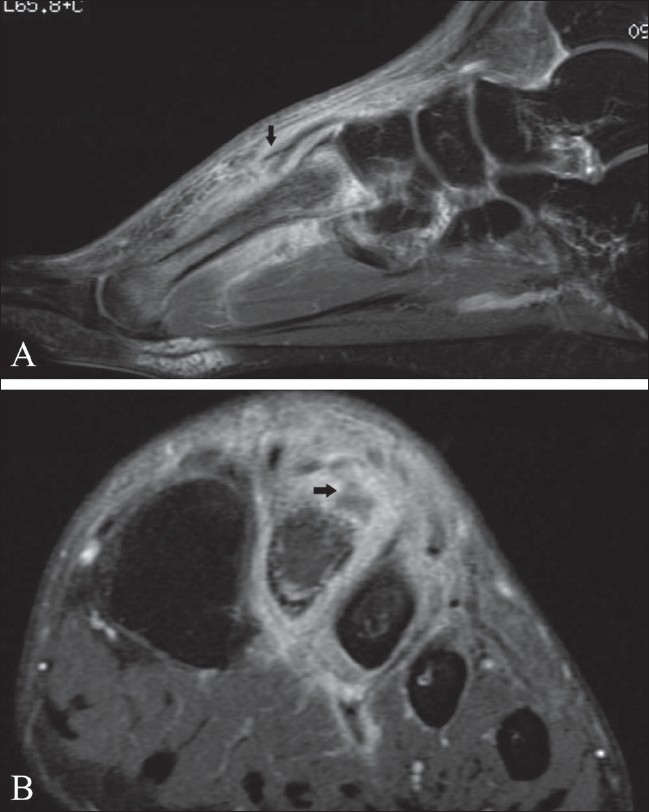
Tuberculous osteomyelitis of the second metatarsal bone of the left foot. Sagittal, fat-suppressed, T2W MRI (A) shows bone marrow edema, adjacent tenosynovitis (arrow) of the extensor tendon and increased signal within the adjacent soft tissues on the plantar and dorsal aspects of the foot. Coronal, contrast-enhanced, fat-suppressed, T1W MRI (B) shows marked enhancement of the soft tissue involvement with central necrosis and abscess formation on the dorsal aspect of the second metatarsal bone (arrow). Note the hazy contour of the dorsal cortex of the diaphysis
